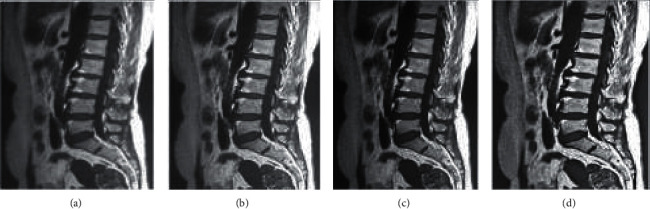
MRI image reconstruction results of lumbar nerves by the three algorithms. (a) Original MRI image of a male patient; (b) image denoised by ICA; (c) image denoised by Fast ICA; and (d) image denoised by M-Fast ICA.
